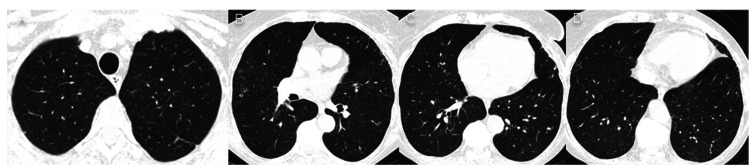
Chest CT 21 months after hospital discharge. Resolution of pulmonary condensations and ground-glass-opacification areas with corticotherapy.
